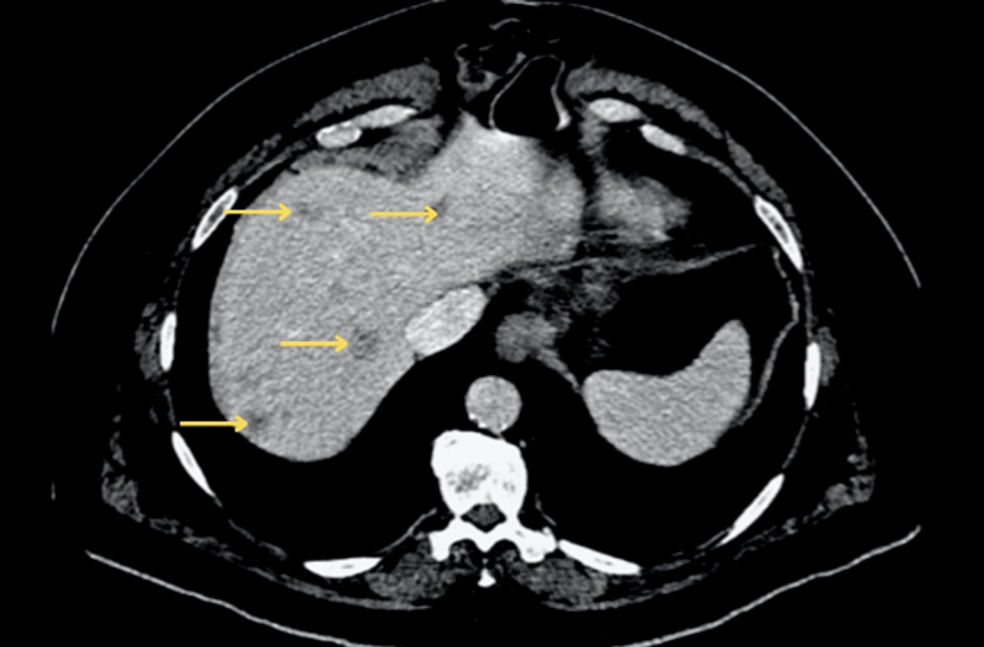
CT scan of the abdomen prior to immunotherapy. Arrows highlight a selection of numerous hypoenhancing liver lesions, indicative of metastasis.
